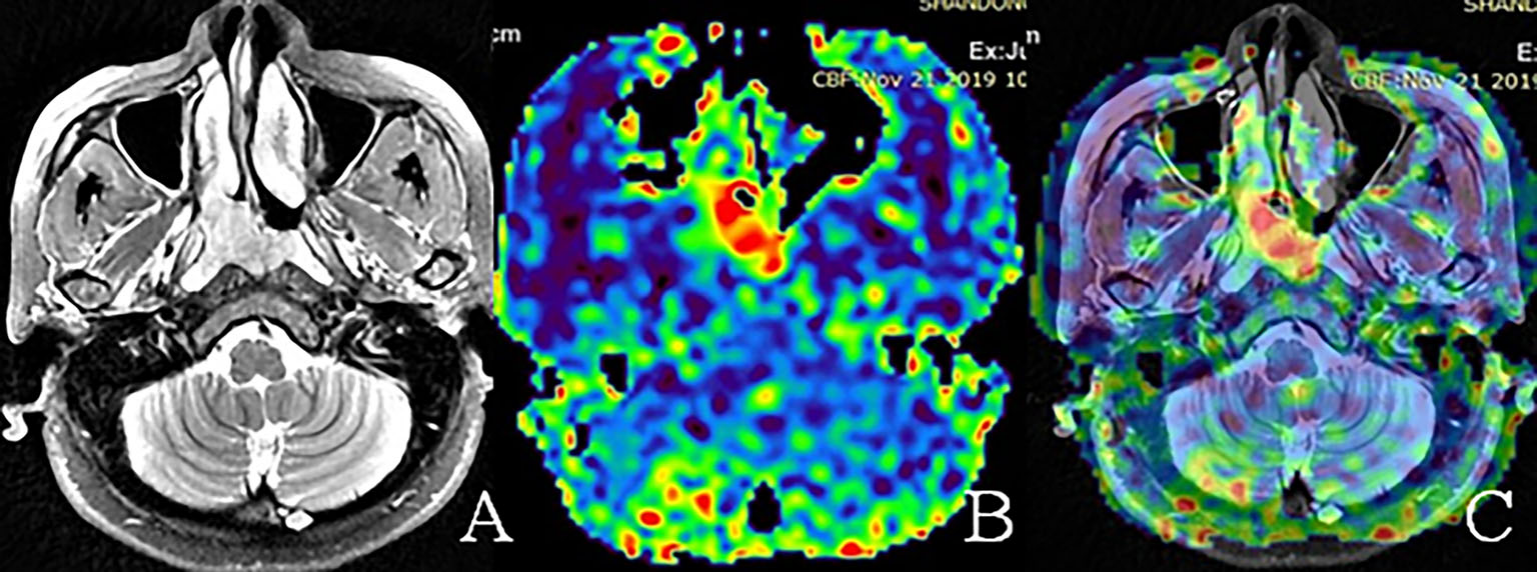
Representative examples of nasopharyngeal tumor region of interest (ROI) on different images. (A) ROI on the axial T2WI. (B) The ROI on the corresponding TBF map, which demonstrated higher perfusion compared with the surrounding tissue. (C) The same ROI on T2WI and ASL fusion images. The images of (A–C) were obtained from the pre-treatment MRI of a 66-year-old woman with a T_3_ non-keratinizing undifferentiated tumor.
